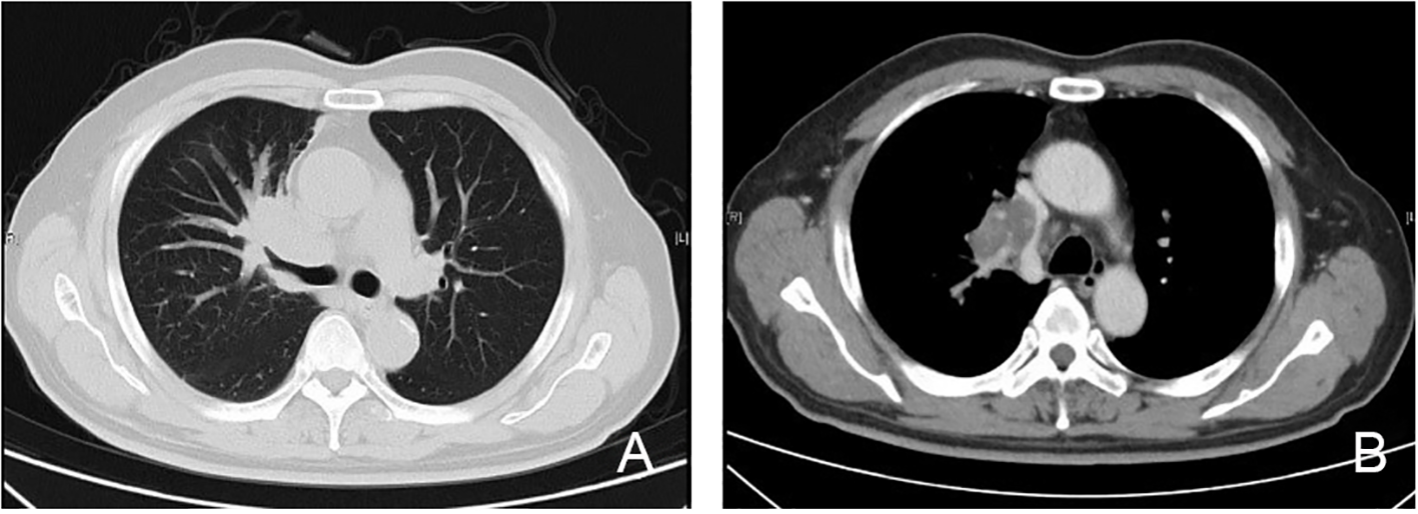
CT imaging of primary lung extranodal NK/T-cell lymphoma (ENKTL). (A) Lung window CT scan shows an irregular mass in the right hilum, with evidence of airway compression and partial obstruction of the bronchus. The mass is lobulated with spiculated borders, consistent with an aggressive lesion, and appears to be close in proximity to the pulmonary vasculature. (B) Mediastinal window CT scan demonstrates enlargement of several mediastinal lymph nodes, suggesting possible lymphatic involvement. The mass appears to be invading adjacent mediastinal structures, raising concerns about local invasion into the surrounding tissues and vascular compression. CT, computed tomography; ENKTL, extranodal NK/T-cell lymphoma; NK, natural killer.
